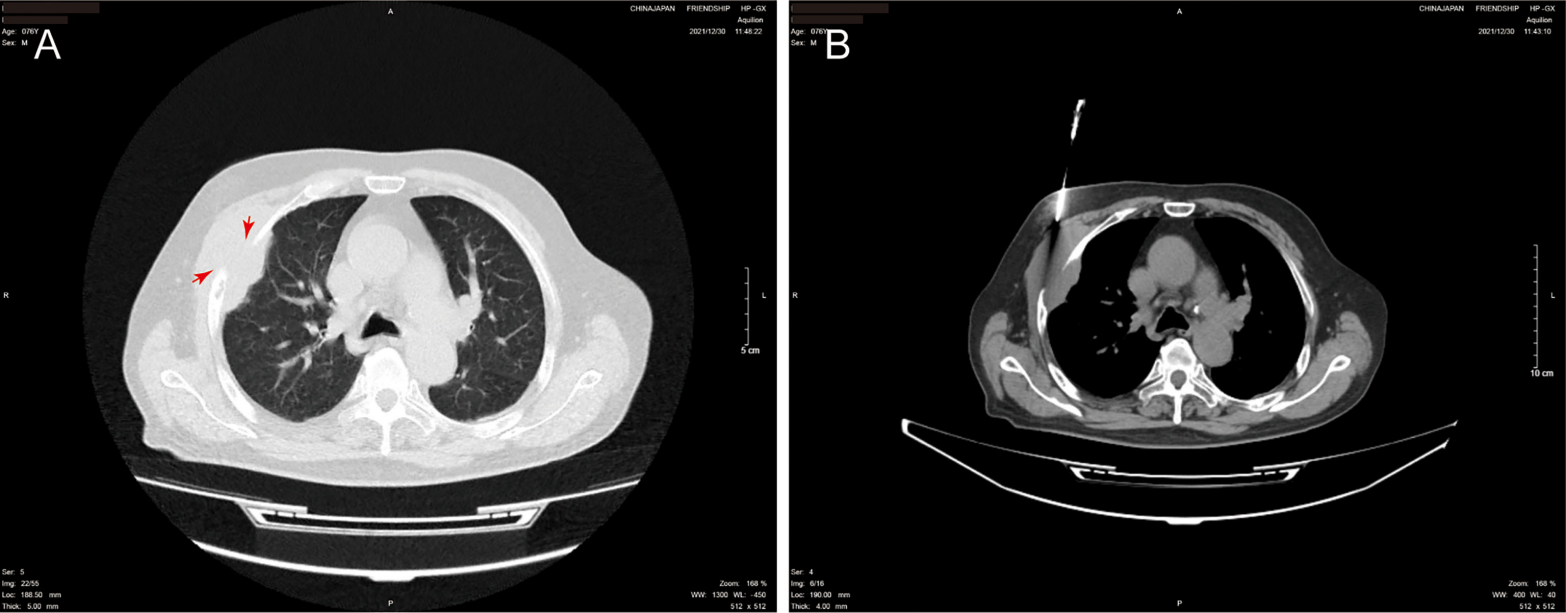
Chest computed tomography (CT) scans. (A) Pulmonary window showing the maximum cross-section of the mass (89 × 42 mm). The image shows the third and fourth ribs, which exhibit insect-like bone destruction; the adjacent pleura is thickened (red arrow). (B) Mediastinal window obtained under CT-guided core needle biopsy.
